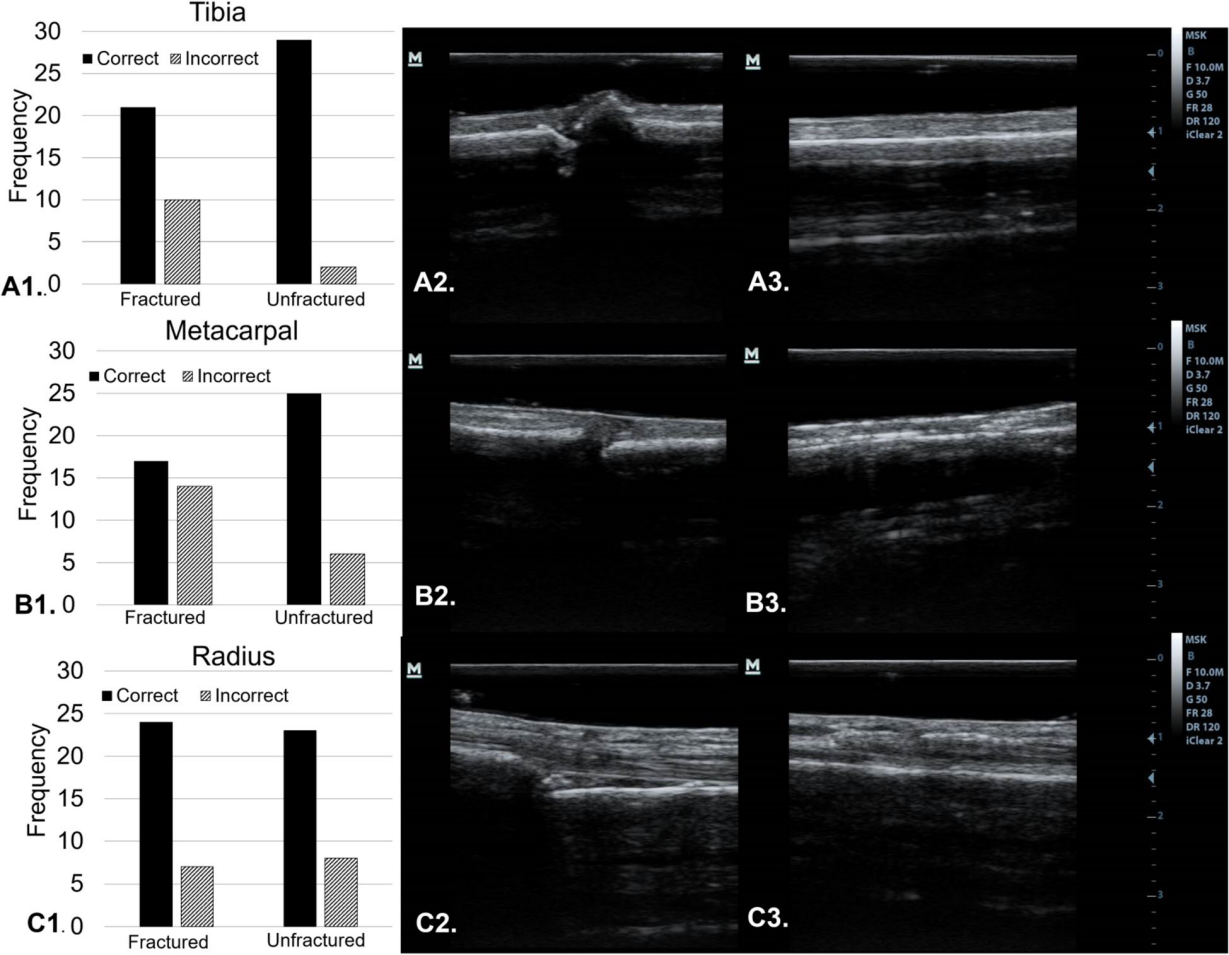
Ultrasound Workshop Testing Stations. Panel A1 depicts participant responses when asked to scan a tibia and determine if it was fractured or unfractured. Panels A2 and A3 show instructor acquired ultrasound images of the fractured and unfractured sides of the tibia testing station cadaver respectively. This same panel organization is conserved when viewing rows B and C of Fig. 4 which depict the data and ultrasound images from the metacarpal and radius stations respectively. The Y axis of panels A1, B1, C1 represent the number of participant responses
